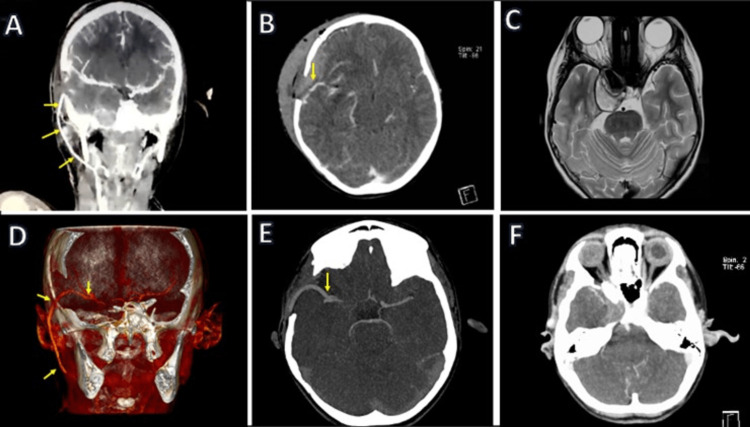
Postoperative images at two months (A–C) and six months (D–F) after surgery. Images (A) and (D) from the coronal view show a patent bypass (arrows). Images (B) and (E) show the CTA in axial view. The MCA's bypass (arrow) and branches are seen without evidence of low-density areas. Images (C) and (F) show pre- and postoperative (six months) images of the aneurysm. The mass effect is reduced with the improvement of the cavernous sinus symptomatology. CTA: computed tomography angiography; MCA: middle cerebral artery
